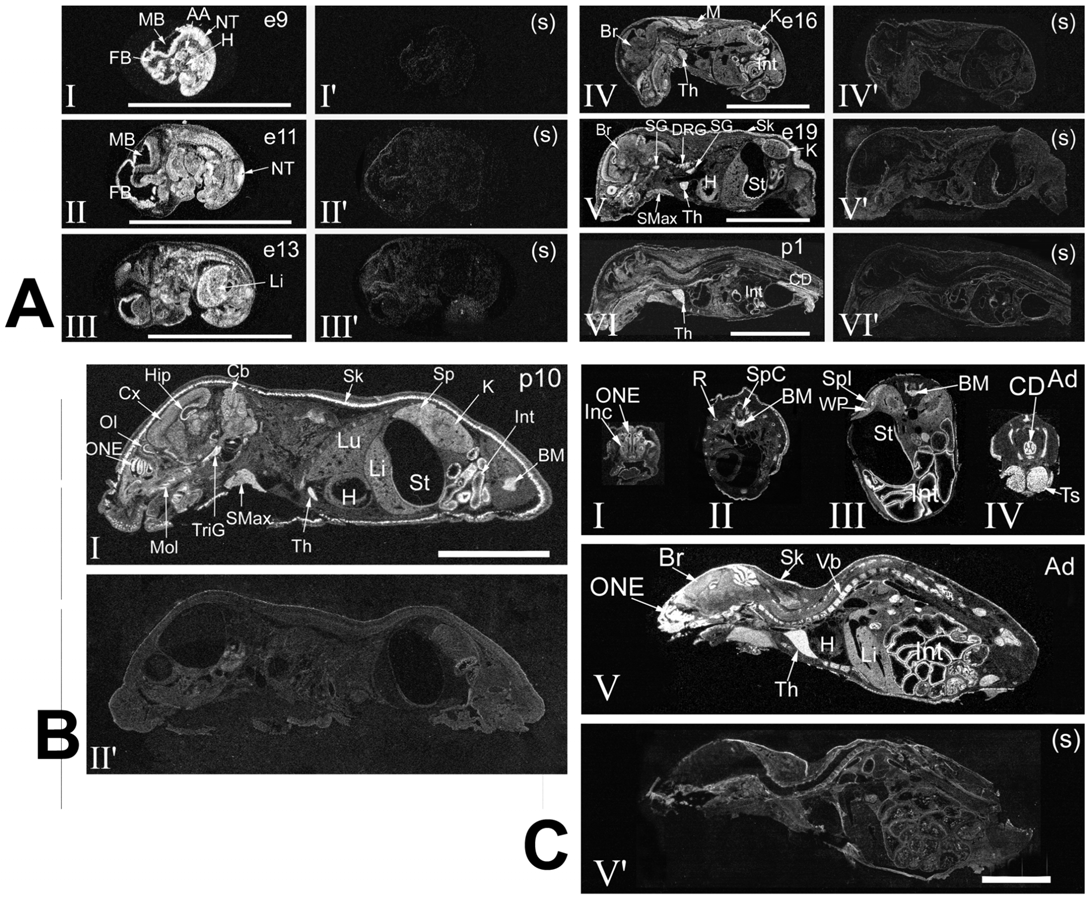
ISH analysis of Pno1 expression during ontogeny. AA: arch artery; Ad – adult; BM: bone marrow; Br: brain; Cb: cerebellum; CD: colon, descending; Cx: cerebral cortex; DRG: dorsal root ganglion; FB: forebrain; H: heart; Hip: hippocampus; Inc: incisors, teeth; Int: intestine; K: kidney; Li: liver; Lg: lacrimal gland; Lu: lungs; M: muscles, striated; MB: midbrain; Mol: molars, teeth; NT: neural tube; OL: olfactory lobe; ONE: olfactory neuroepithelium; R: rib; SG: sympathetic ganglion; Sk: skin; SMax: submaxillary gland; SpC: spinal cord; Spl: spleen; St: stomach; Th: thymus. TriG; trigeminal ganglion; Ts: testes; Vb: vertebrae. Bars = 1 cm. (s): sense cRNA used as a probe. The bars = 1 cm. Darkfield x-ray film autoradiography is shown. *A. Pno1 expression from embryonic day 9 (e9) to post-natal day 1 (p1)*. Panels I–VI: anti-sense cRNA as probe. Panels I’–VI’: sense cRNA as probe. *B. Pno1 expression on p10.* Panel I: anti-sense cRNA as probe. Panel I’: sense cRNA as probe. *C. Pno1 expression in adulthood*. Panels I–V: anti-sense cRNA as probe. Panel V’: sense cRNA as probe.
